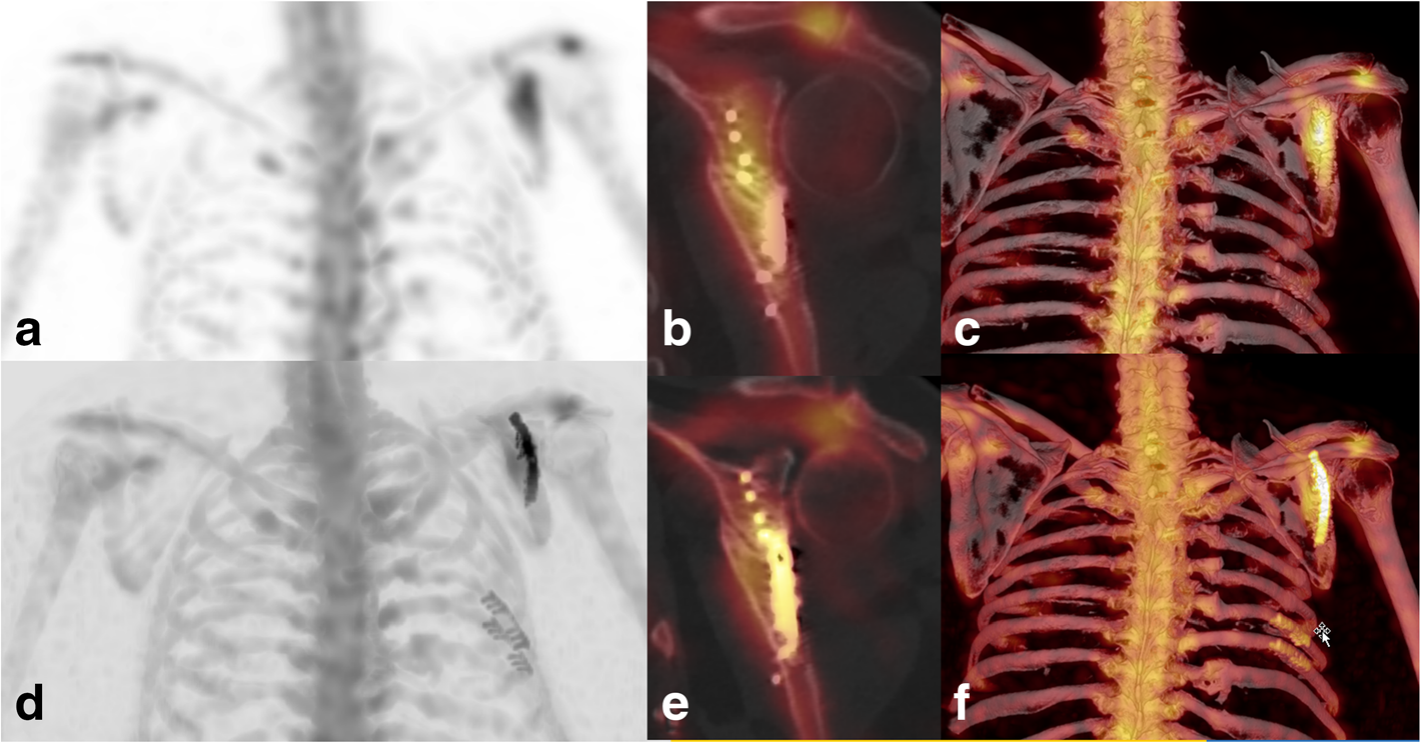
xSPECT Bone “shining metal artefact” in the shoulder. SPECT/CT images of a 57-year-old male patient with pain in the left shoulder 3 years after osteosynthesis of a scapula fracture and two ribs. MIP image with xSPECT Bone reconstruction (d), fused coronal SPECT/CT (e) and fused 3D reconstruction (f) show high “pseudouptake” of the plates and screws in the scapula and increased uptake around the non-union. “Pseudouptake” of the screws and plate in the ribs. Corresponding images (a–c) with conventional Flash3D reconstruction show no increased uptake of the radiotracer around the screws but active remodelling in the adjacent scapular bone around the non-union and no uptake in the ribs. Besides non-union, the patient suffered from neuropathic pain and conservative therapy was initiated
